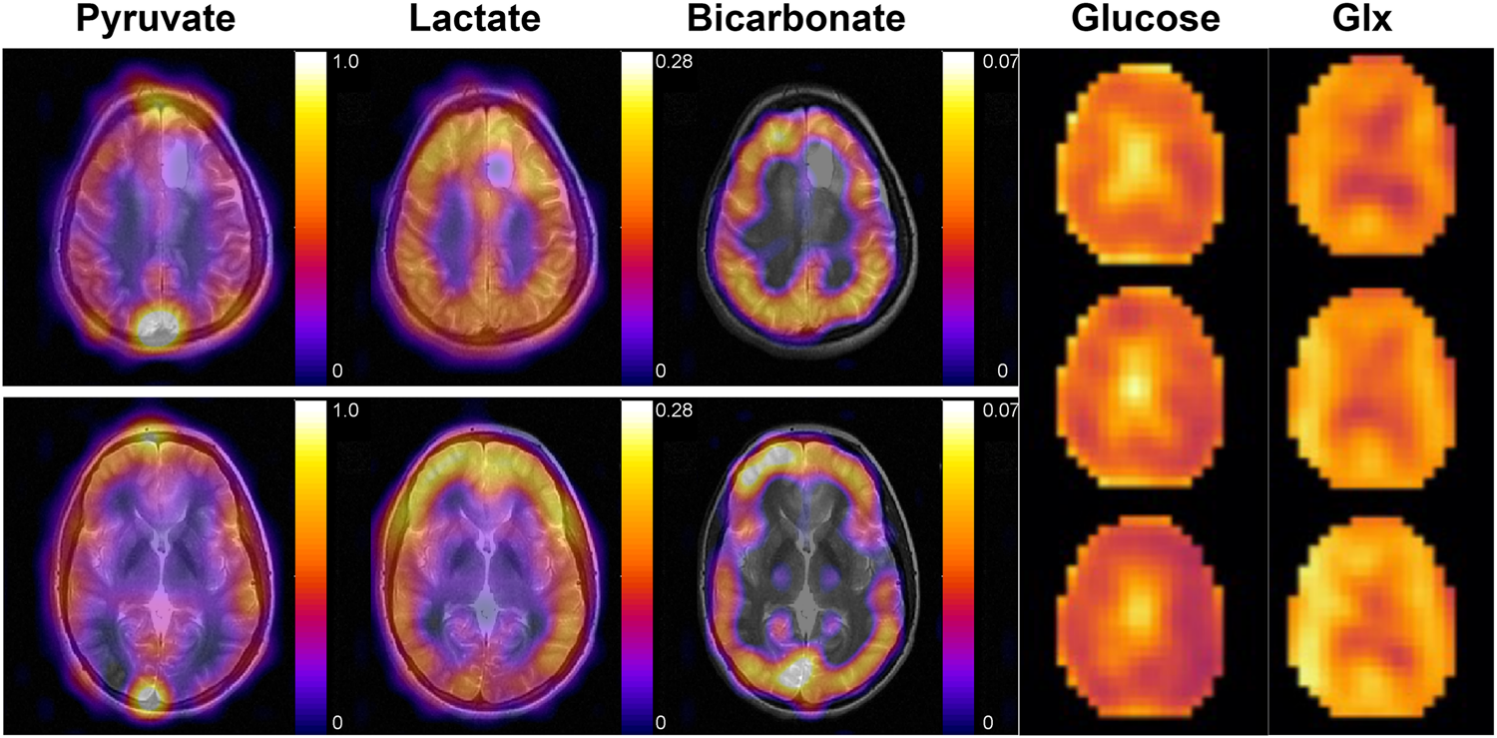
^13^C MR images, acquired at 3 T, of hyperpolarized ^13^C-labelled pyruvate, lactate and bicarbonate (overlaid on a T_2_-weighted ^1^H image) and ^2^H MR images, acquired at 9.4 T, of ^2^H-labelled glucose and Glx in the brains of healthy volunteers. The ^13^C images are the sum of 20 images acquired over 60 s following the intravenous injection of 0.43 mL/kg of ~250 mM hyperpolarized [1-^13^C]pyruvate. The nominal image resolution was 1.5 × 1.5 × 2 cm^3^. The ^2^H images show three 10 min images, acquired between 91.5 and 121.5 min after oral administration of 0.75 g/kg body weight of [6,6’-^2^H_2_]glucose. The nominal image resolution was 1.5 × 1.54 × 1.29 cm^3^. Bicarbonate and Glx labelling are higher in the grey matter, reflecting increased TCA cycle activity in grey matter as compared to white matter. The images were taken, with permission, from^49,50^.
